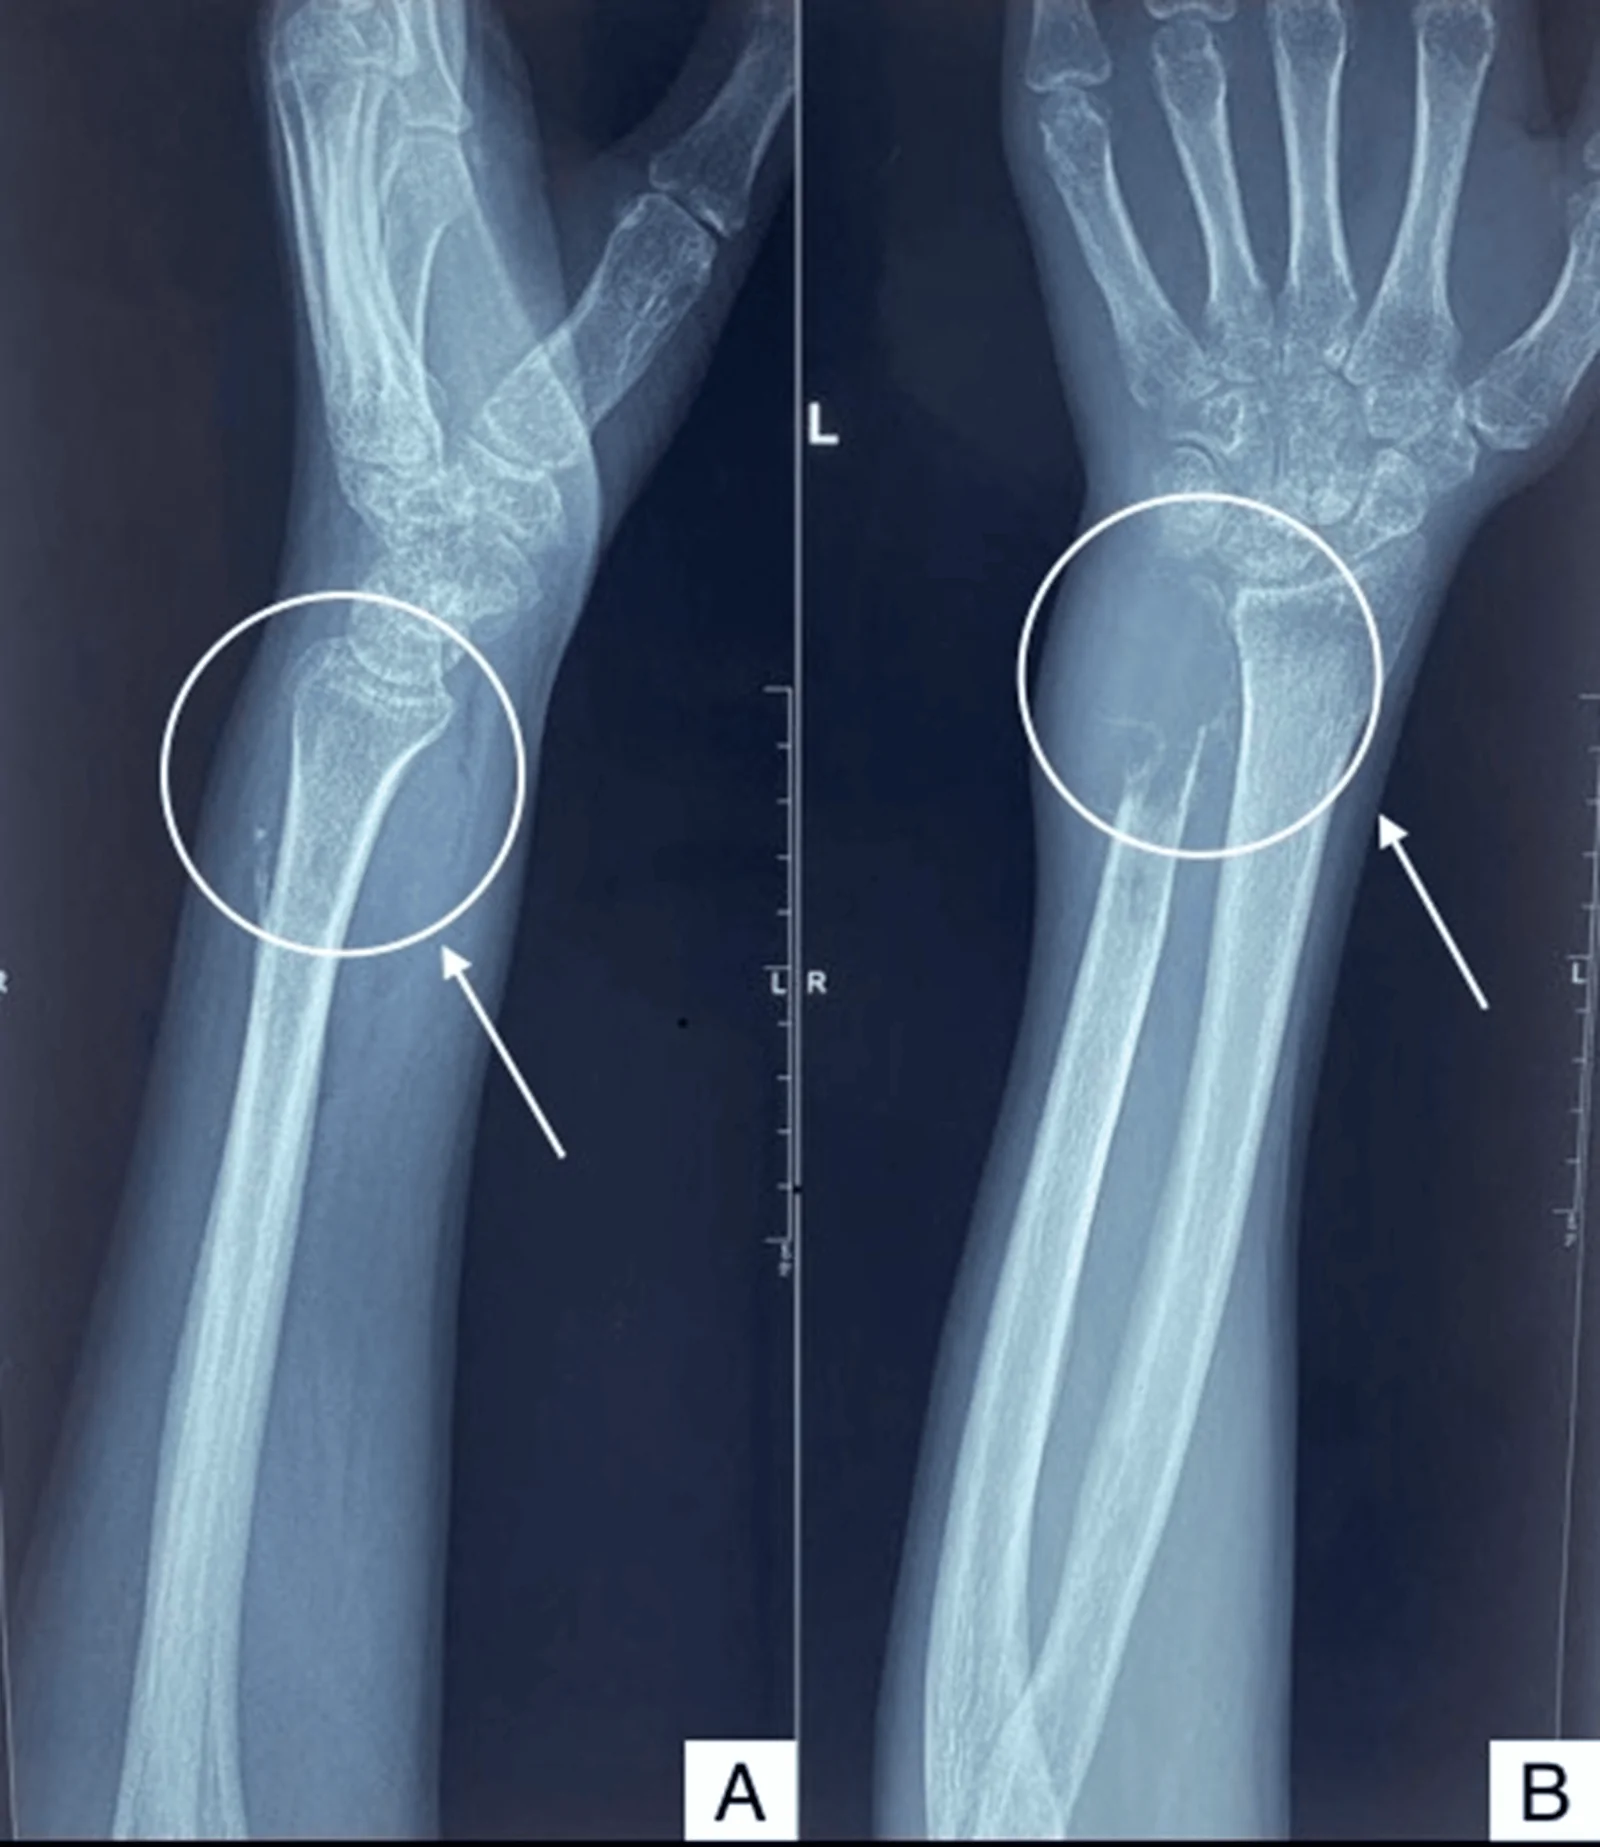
(A-B) Preoperative radiograph demonstrating an eccentric expansile lytic lesion involving the distal ulna.
